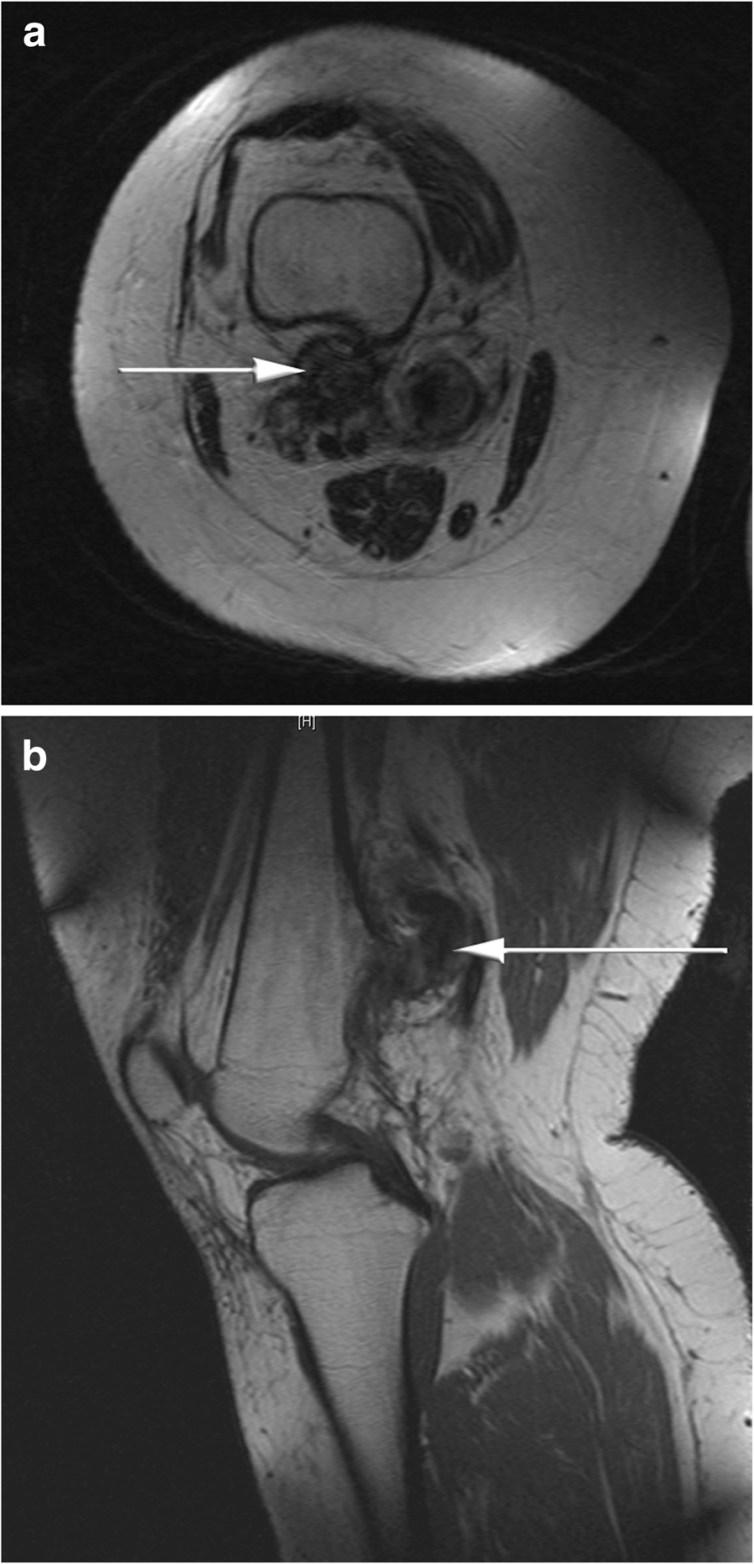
MRI of the right distal femur. a. MRI (T2-TSE-TRA-512) depicting a soft tissue component being pierced by sharp exostosis tip (axial view); white arrow points to soft tissue mass. b. MRI (T1-SE-SAG-512) depicting a soft tissue component around sharp exostosis tip (sagittal view); white arrow points to soft tissue mass.
